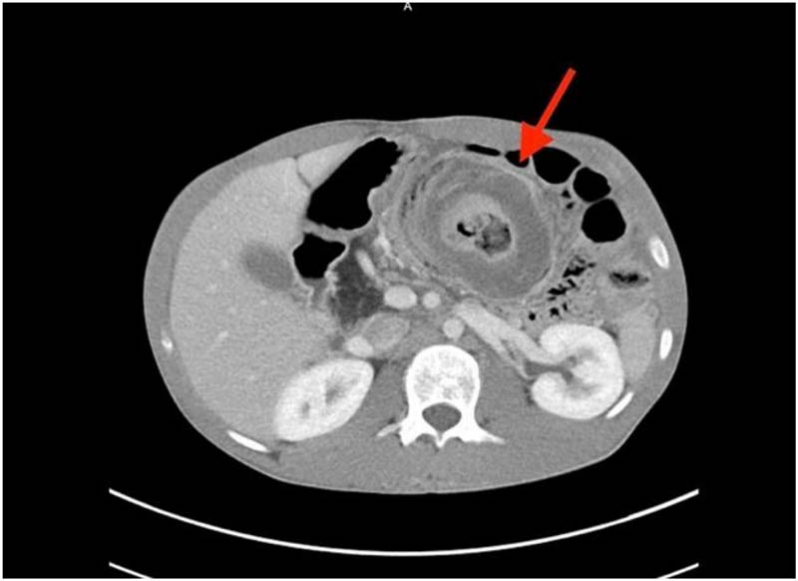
Axial CT scan showing characteristic target sign of bowel intussusception.
